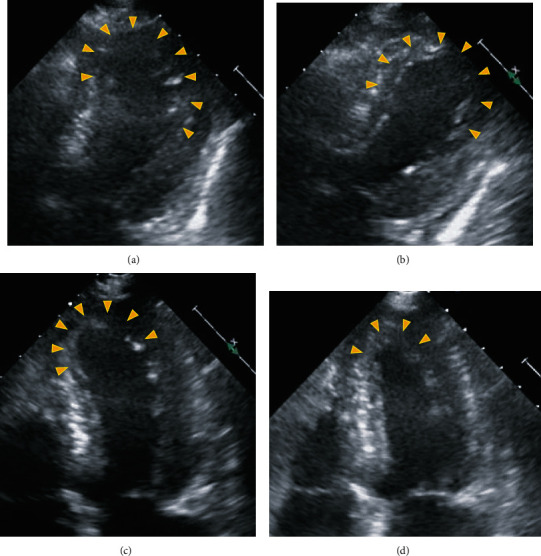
Echocardiogram shows gradual improvement of left ventricular systolic dysfunction: (a) admission (day 0), (b) hospital day 2, (c) day 5, and (d) day 9. Yellow arrow indicates an area of abnormal wall motion in end-systole.
